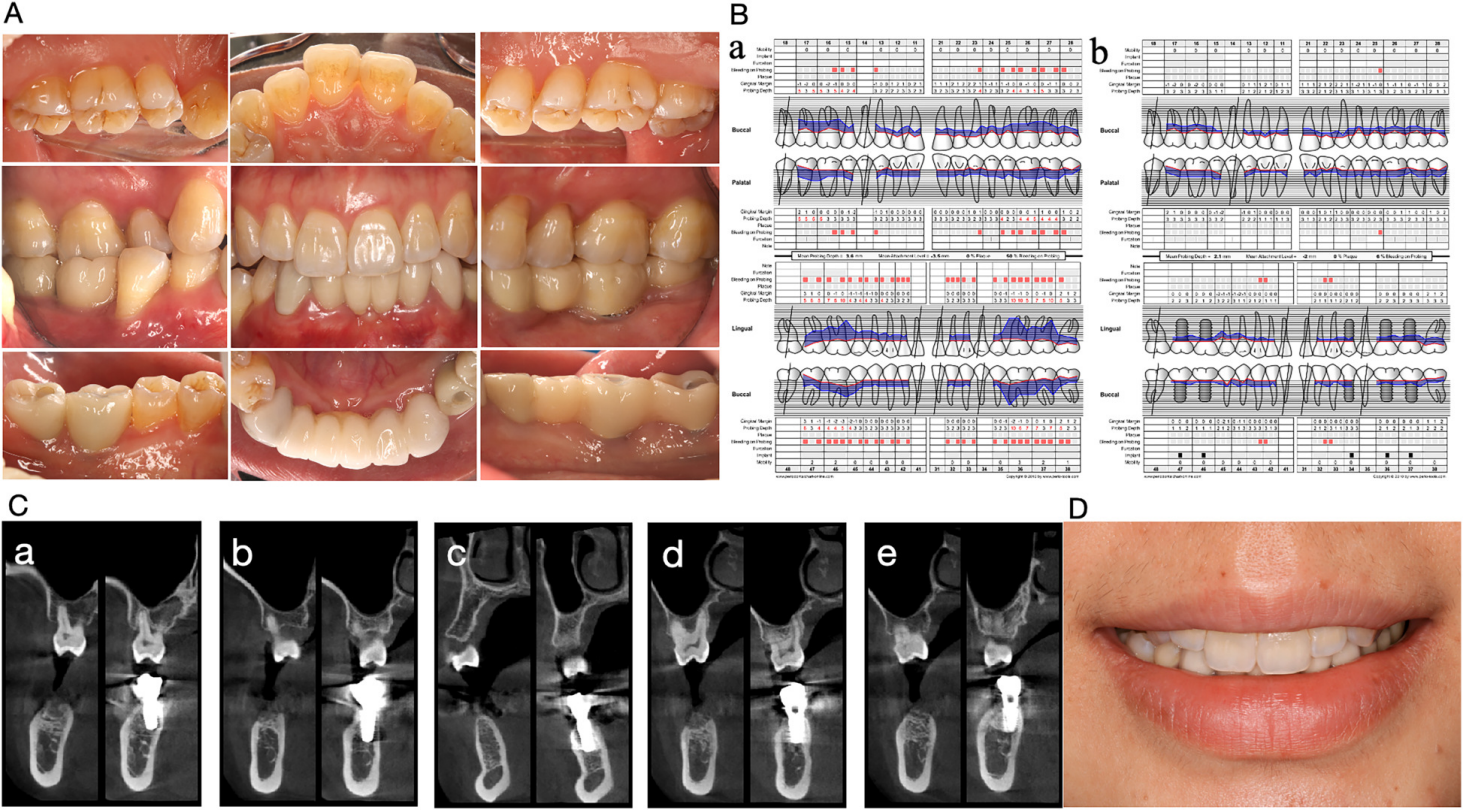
Oral condition of the Case 1 patient two years after implant restoration. (A) Intraoral photo. (B) Comparison of periodontal parameters. (a) Initial visit. (b) Two years after implant restoration. (C) CBCT comparison before and two years after implantation. From (a) to (e), the mandibular implants from right to left in case1 patients are shown before and two years after implantation. Implants were tightly integrated with the alveolar bone and the bone height was stable. (D) Frontal smiling photo.
